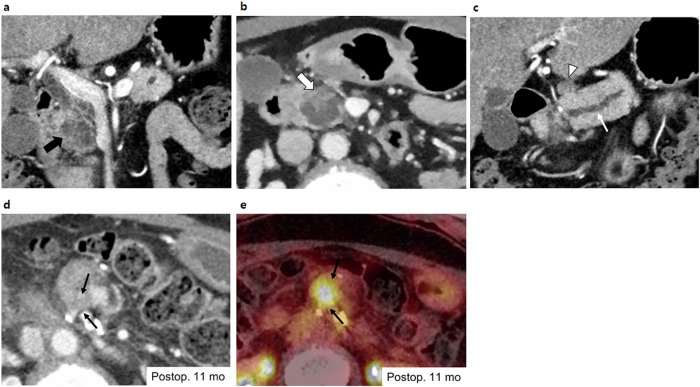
Another representative case of a 57-year-old female patient who underwent surgery for IPMN. a On the preoperative CT, there was a 3.2 cm pleomorphic cystic lesion in the pancreas head (thick black arrow). b The lesion had about 1.8 cm EMN (thick white arrow). c The MPD (white arrow) was mildly dilated to 6 mm. A prominent peripancreatic lymph node (arrowhead) was observed; however, as the short diameter was less than 10 mm, it was not considered lymphadenopathy. After surgical resection, the lesion was confirmed to be IPMN with an associated invasive carcinoma, and 11 metastatic lymph nodes were identified. d During postoperative follow-up, a hypoenhancing soft tissue lesion developed around the pancreaticojejunostomy site (black arrow) 11 months after surgery, which was reported to be a tumor recurrence. e This was confirmed by increased F-18 fluorodeoxyglucose uptake (black arrow) on PET/CT
